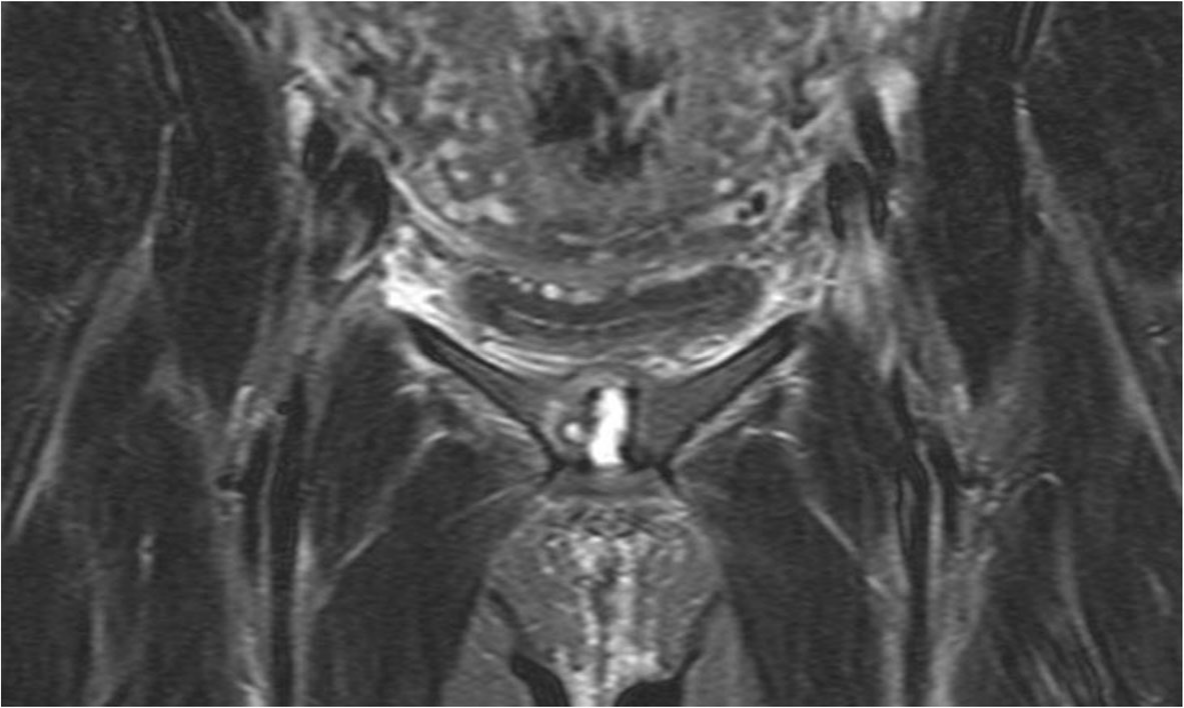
Coronal plane MRI showing oedema in the distracted symphysis with a cavity in the right periarticular subchondral bone, indicating a spread of infection from the cartilaginous disc
